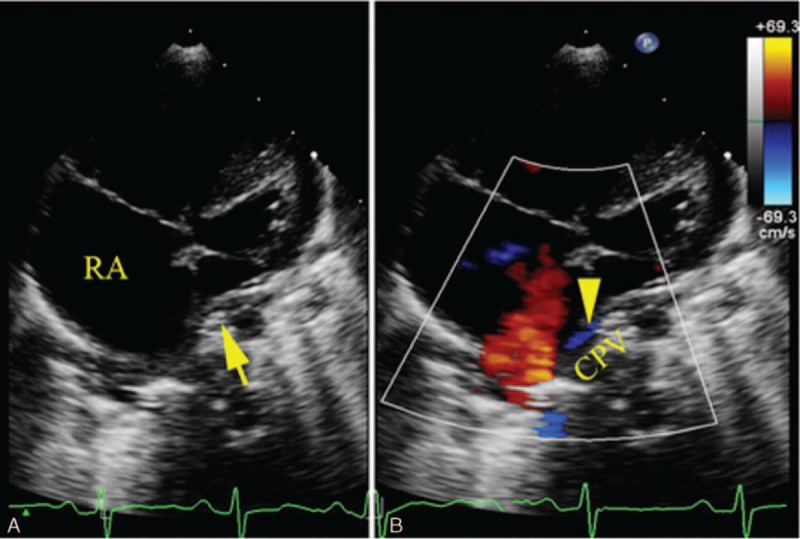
A 6-month-old boy with common pulmonary vein (CPV) draining into the right atrium (RA). (A) Apical 4-chamber view shows septum primum malposition. (B) Color Doppler shows blood flow from CPV to the RA.
